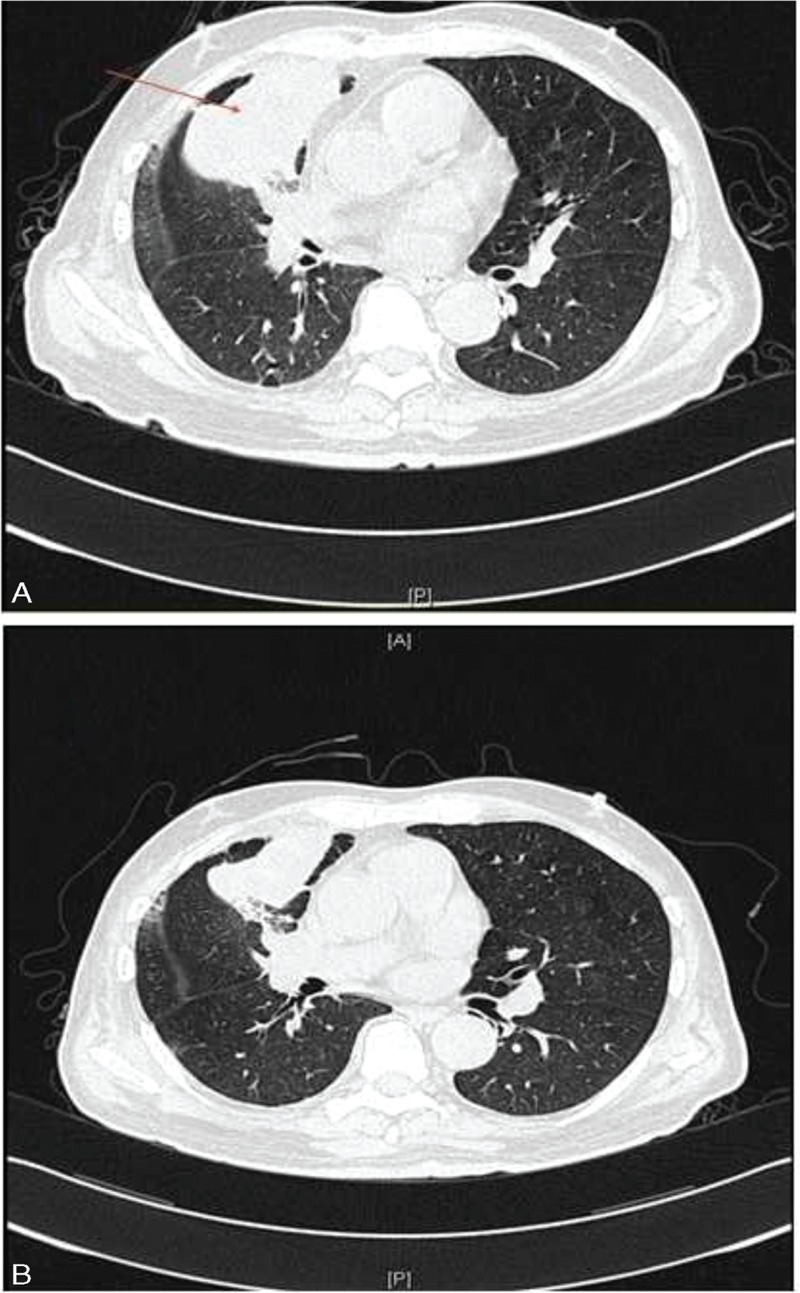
CT shows that mass in right upper lobe (A); mass is smaller after 3 month of apatinib treatment (B). CT = computed tomography.
